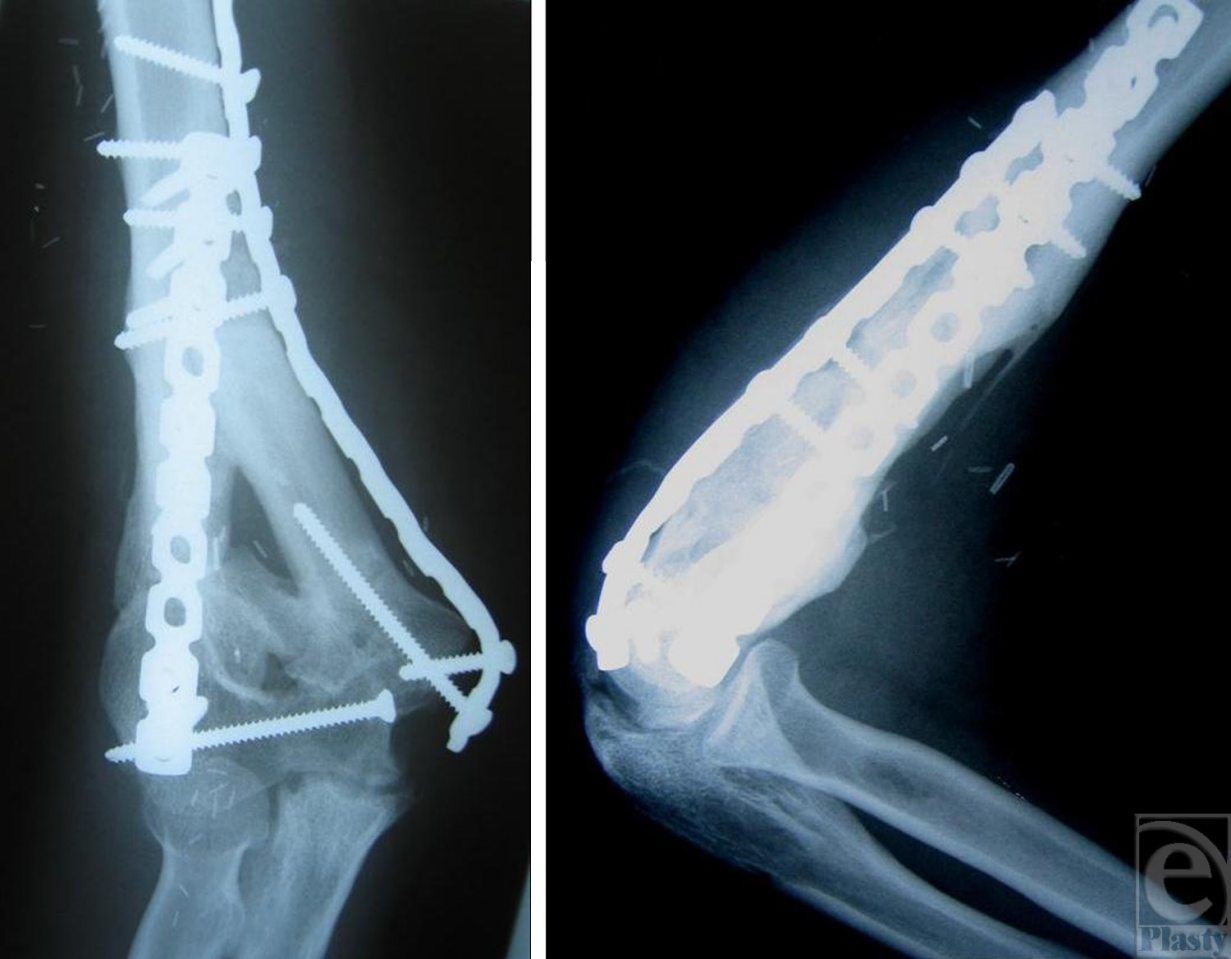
Anteroposterior (left) and lateral (right) radiographs of the right elbow 26 months after transplantation of a 6-cm free vascularized fibular graft. Complete incorporation of the graft and fracture healing are visible.
